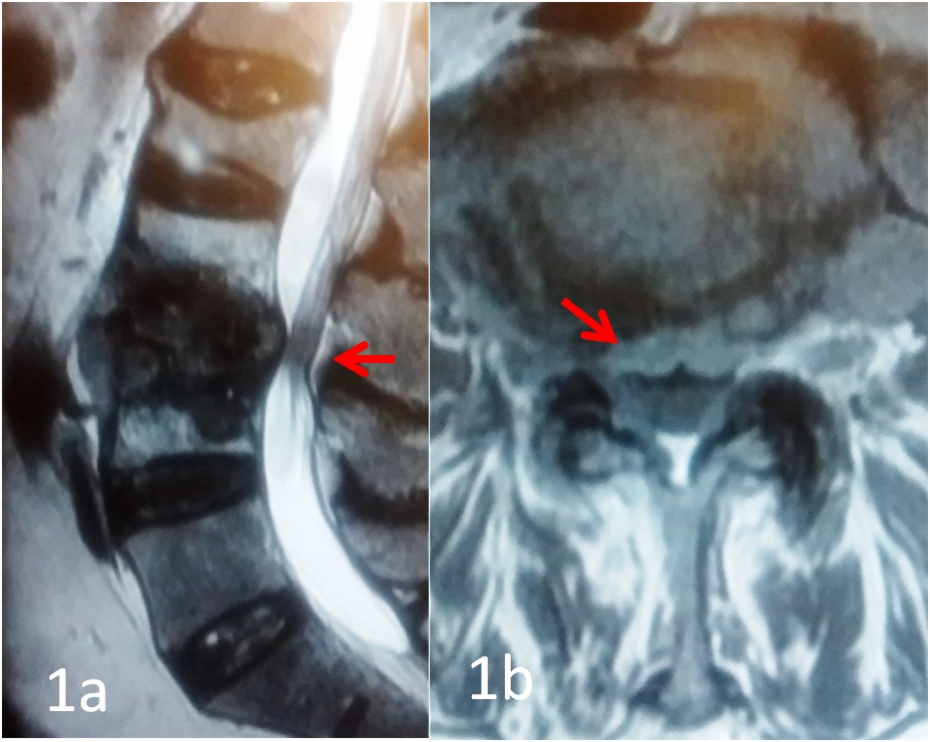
T2W sagittal and axial MRI scans showing L3-4 spondylodiscitis with compression of the thecal sac T2W sagittal and axial magnetic resonance imaging (MRI) scans showing L3-4 spondylodiscitis with compression of the thecal sac (red arrow)
